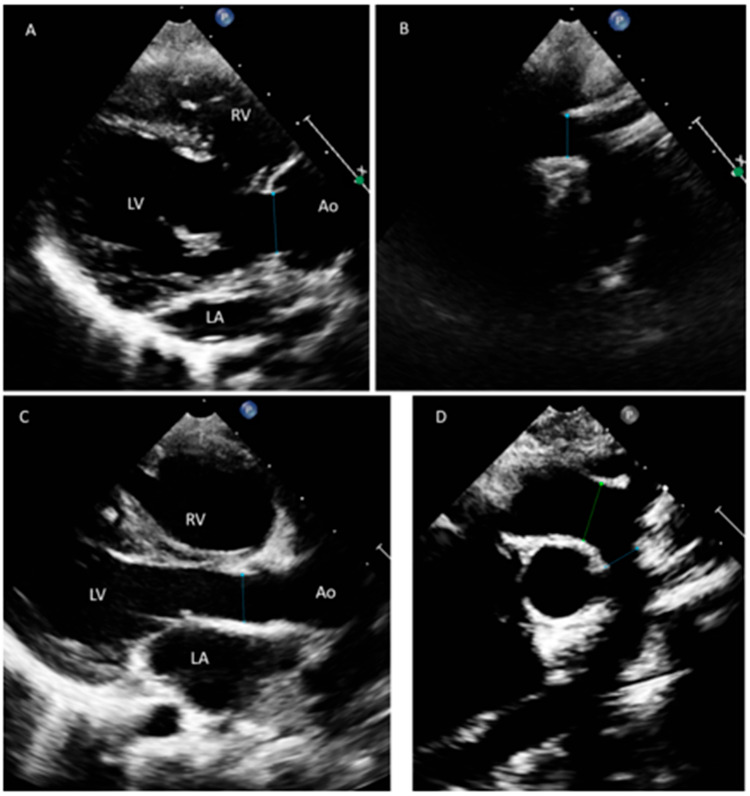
Echocardiographic views taken for both Saudi siblings at the parasternal long-axis views (A,C) and suprasternal sagittal views (B,D). Both siblings have normal measurement and z score for the aortic valve and aortic arch. The first sibling has normal aortic valve (A) that measures 1.4cm (z score 0.3) and normal transverse aortic arch 1.2cm (z score 0.3) (B). The other sibling has normal aortic valve (C) that measures 1.0cm (z score -1) and normal transverse aortic arch 0.8cm (z score—1.4) (D). Ao; aorta, LA; left atrium, LV; left ventricle, RV; right ventricle.
